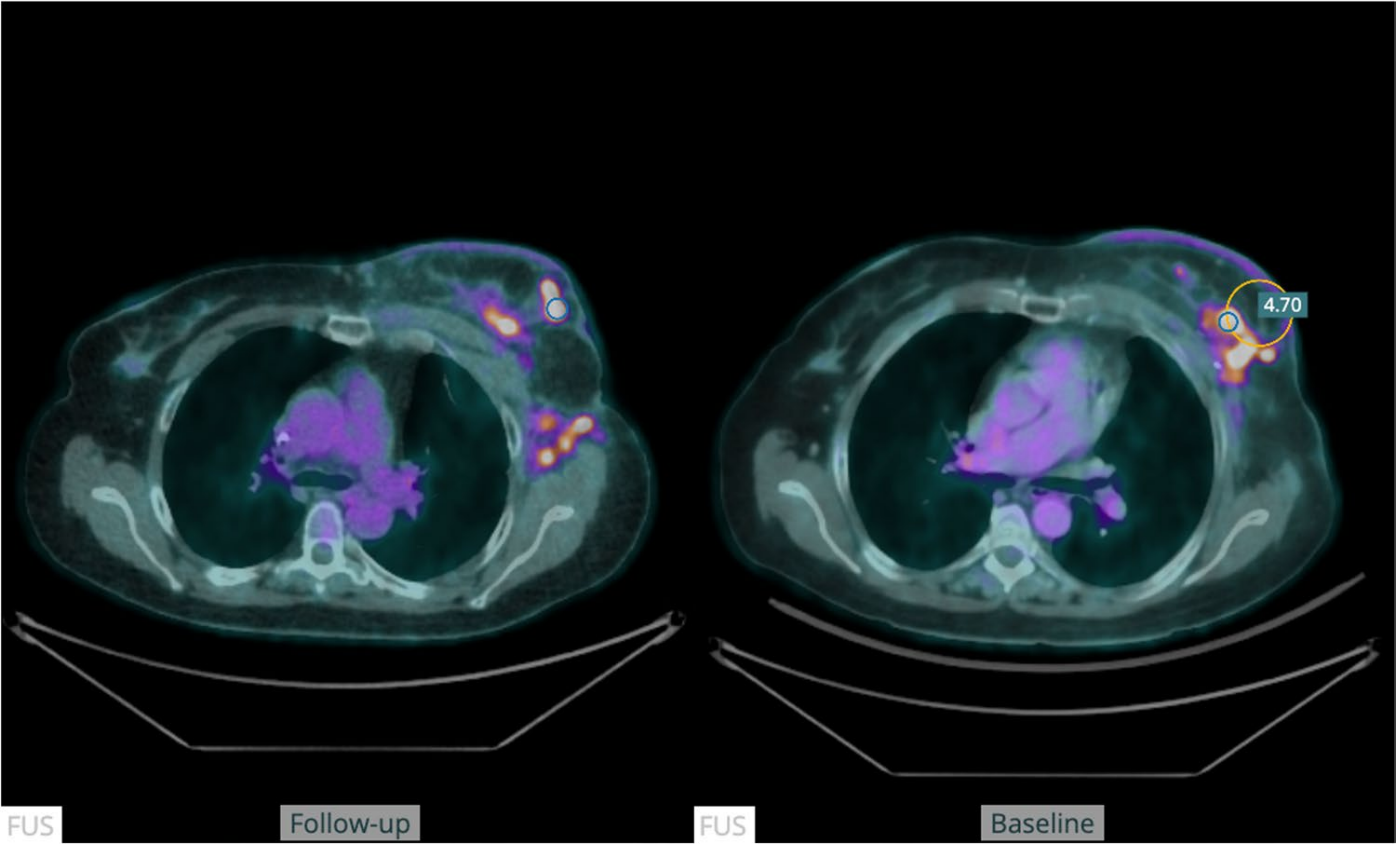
The figure shows a fused transversal slice of a PET-CT scan at baseline (right) and at follow-up (left) in a patient with mixed response in different locations. The blue circle in the follow-up scan represents the location of the SULpeak of the lesion. The yellow circle in the baseline scan represents the”search volume” for the lesion pairing. In this case, the lesion pairing was not accurate
